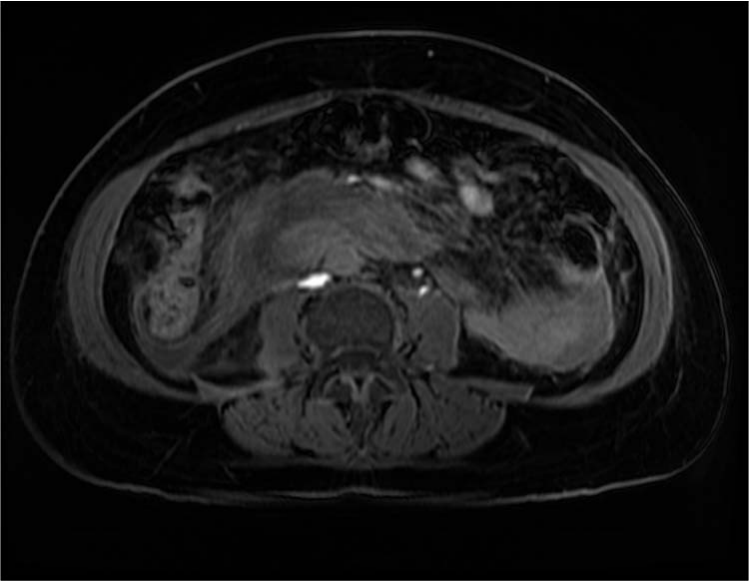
MRI abdomen, axial view. T2 hypointense, T1 iso to hyperintense material surrounding D2/D3 without enhancement in keeping with hemorrhage. No vascular or bowel wall lesion identified.
